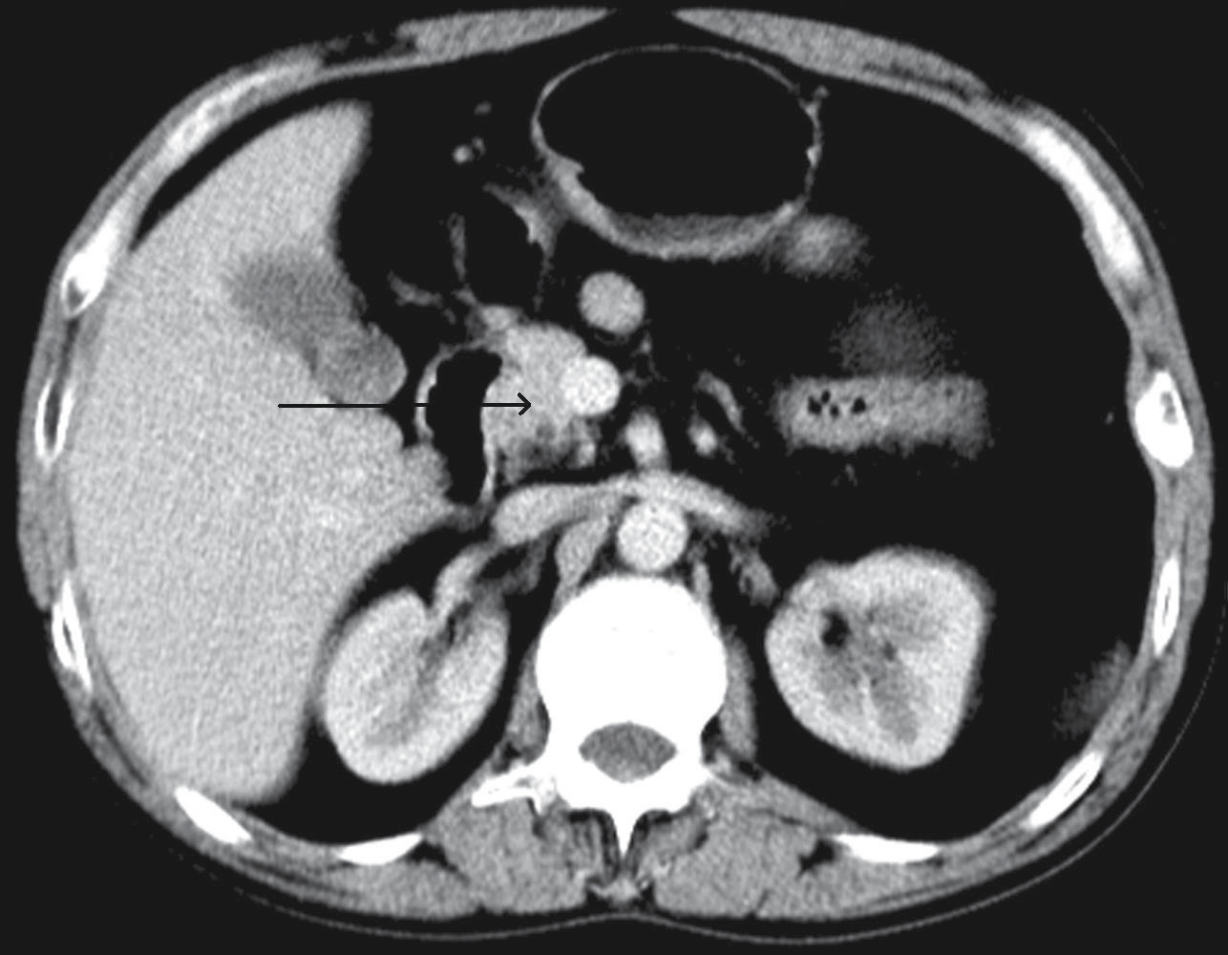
Axial contrast-enhanced CT image shows absence of neck, body, and tail of the pancreas; there is a truncated head (black arrow)
